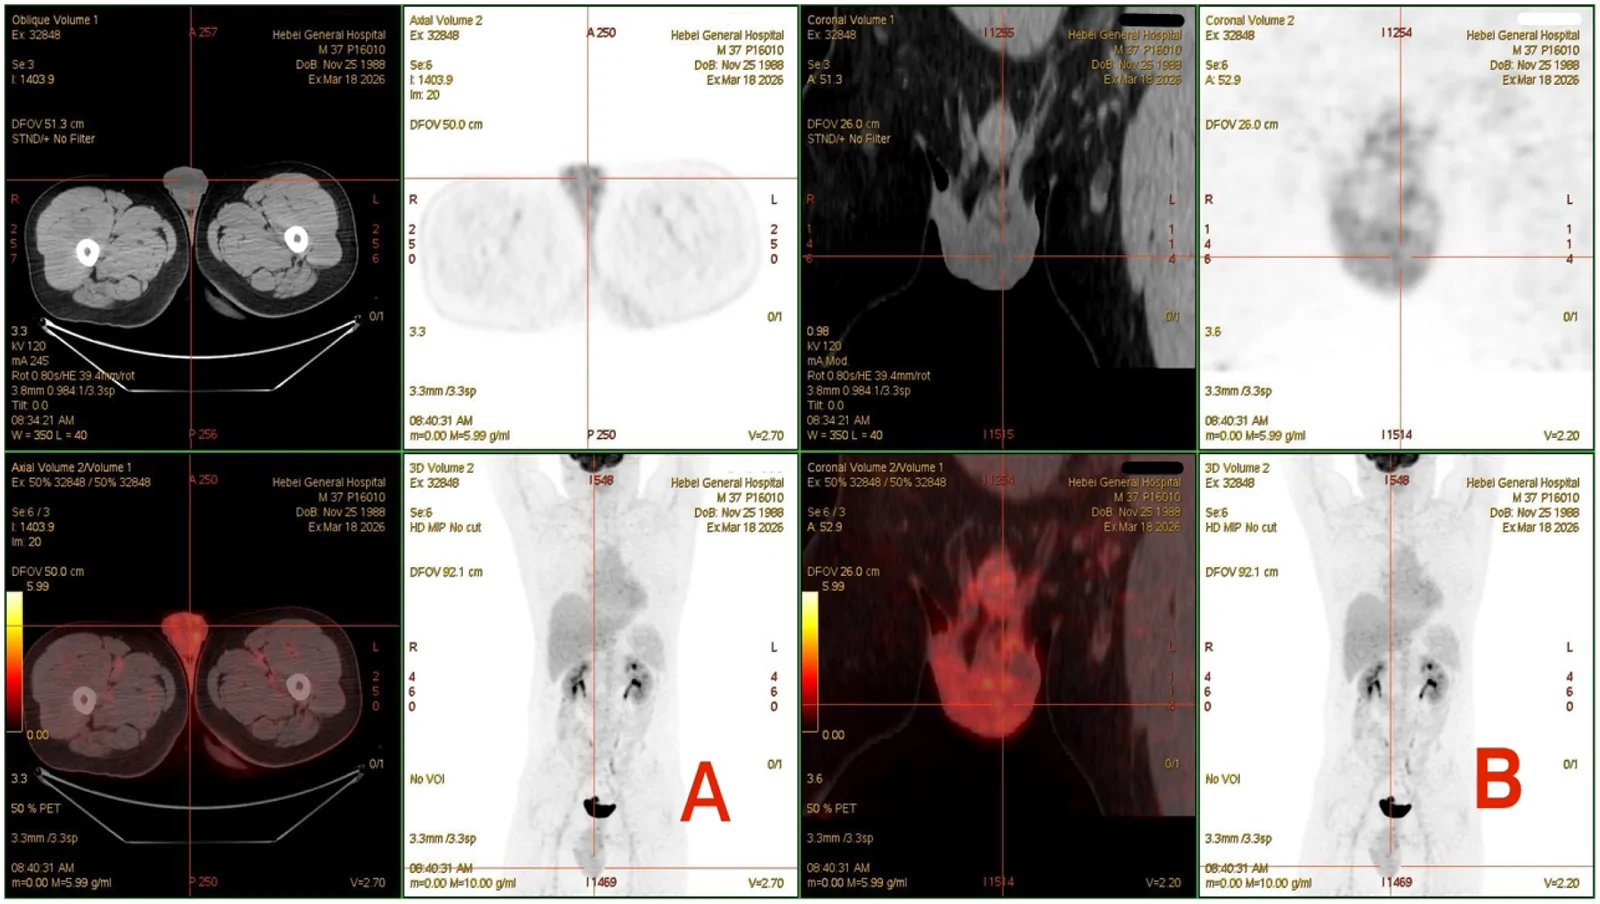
Positron emission tomography/computed tomography (PET/CT) imaging of the scrotal lesion. (A) Axial views encompassing unenhanced CT (upper left), PET (upper right), fused PET/CT (lower left), and a whole-body maximum intensity projection (MIP) image (lower right). The cross-sectional images clearly demonstrate an enlargement of the left hemiscrotum characterized by increased internal soft tissue density when compared to the normal contralateral side. This structurally abnormal region exhibits mild metabolic activity on the corresponding PET and fused images. (B) Coronal views displaying unenhanced CT (upper left), PET (upper right), fused PET/CT (lower left), alongside the whole-body MIP image (lower right). These longitudinal sections further characterize the craniocaudal extent and morphological boundaries of the left scrotal soft tissue lesion, confirming the localized mild metabolic uptake.
